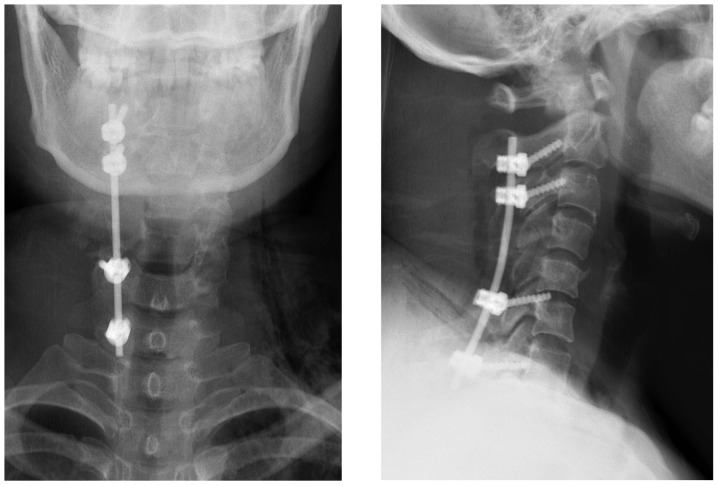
Post-operative anteroposterior and lateral radiographs.
